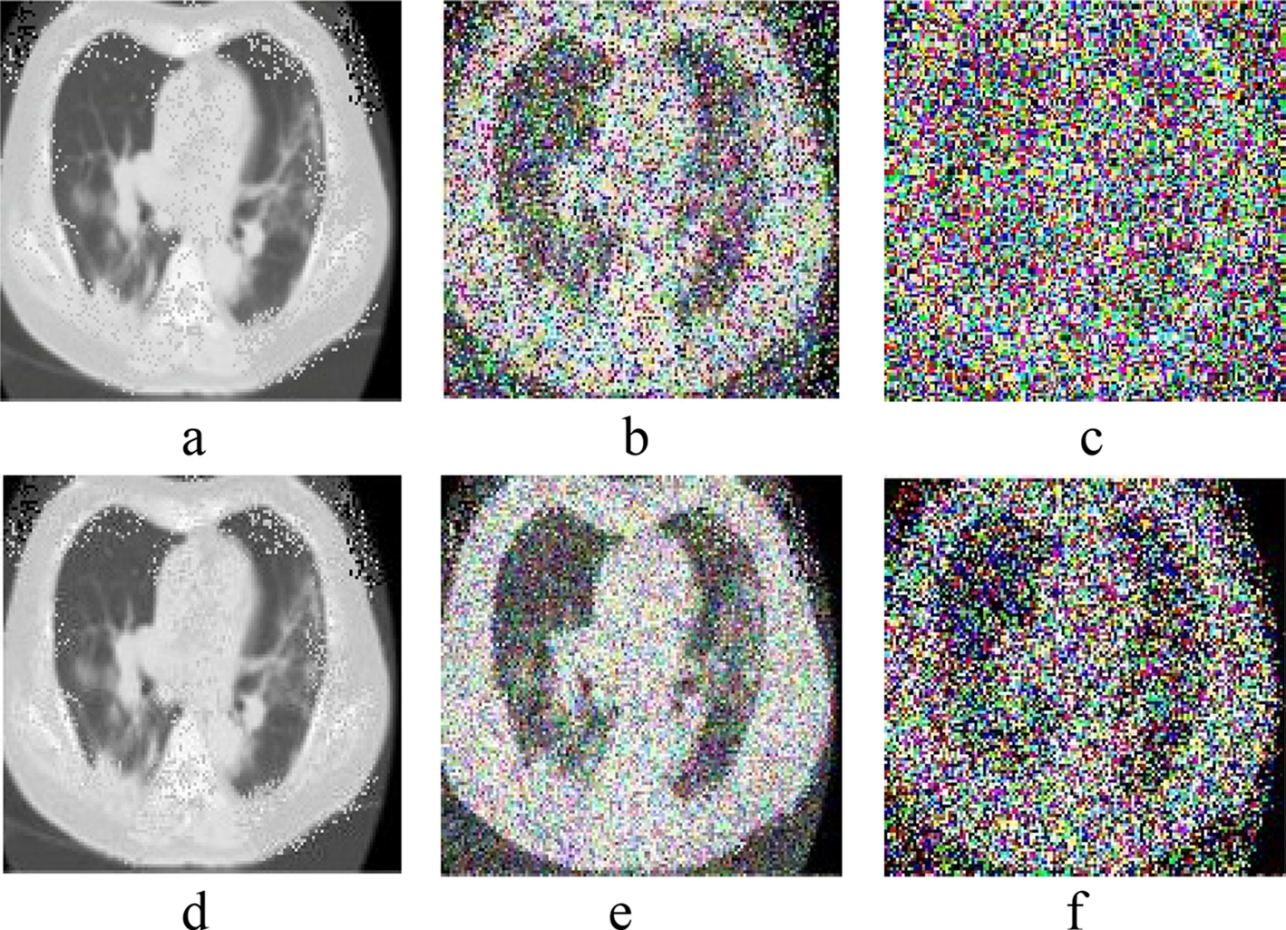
Shows the normal and noisy CT images. (a) normal CT image having natural Impulse noise (b) Impulse noise corrupted image with 50% noise density (c) Impulse noise corrupted image with 80% noise (d) Poisson noise corrupted image with 50% noise density (e) Poisson noise corrupted image with 80% noise density (f) contaminated image with both Impulse and Poisson noise with 80% noise density.
